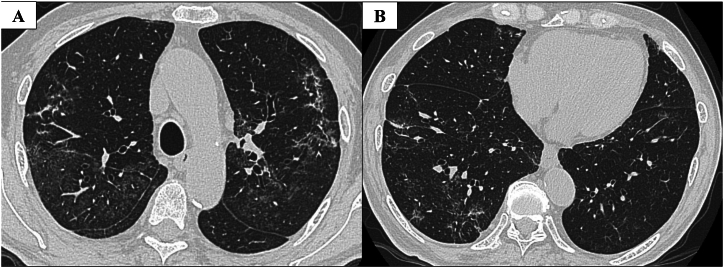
HRCT after 31 days from antigen avoidance test (A) The ground glass opacities in the bilateral upper lobes were improved. (B) The ground glass opacities in bilateral lower lobes were also improved.
